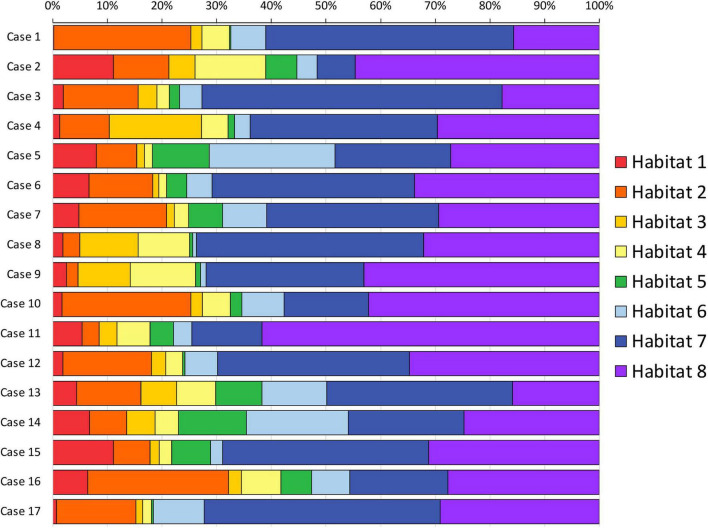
Habitats volumetric representation. The relative volumetric representation of each habitat (expressed as a percentage of the whole FLAIR-tumor volume) is reported for each case included in the series.
